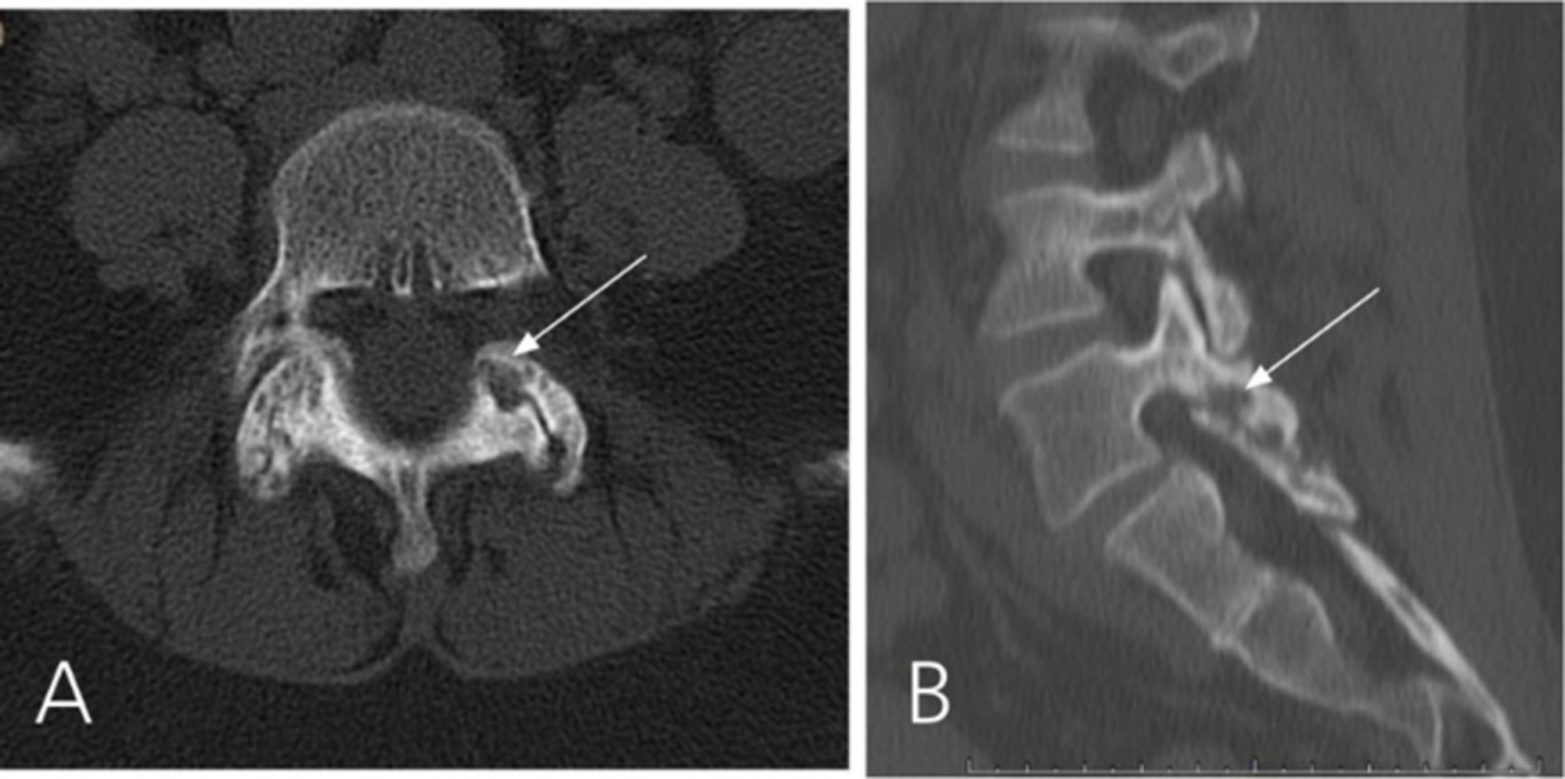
CT scan of Case #1 CT scan showing left L5-S1 facet erosive bony changes (white arrows) suggestive of a septic arthritis of the facet joint. A: axial image. B: sagittal image.
